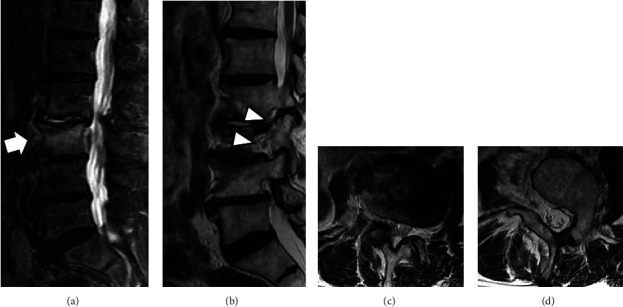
Preoperative magnetic resonance image. (a) STIR midsagittal, (b) T2-weighted right parasagittal, and T2-weighted axial ((c) L2-L3 disc level; (d) L3 pedicle level). White arrow: intensity change due to a L3 fracture. Arrowheads: right L2 (upper) and L3 (lower) nerve root.
